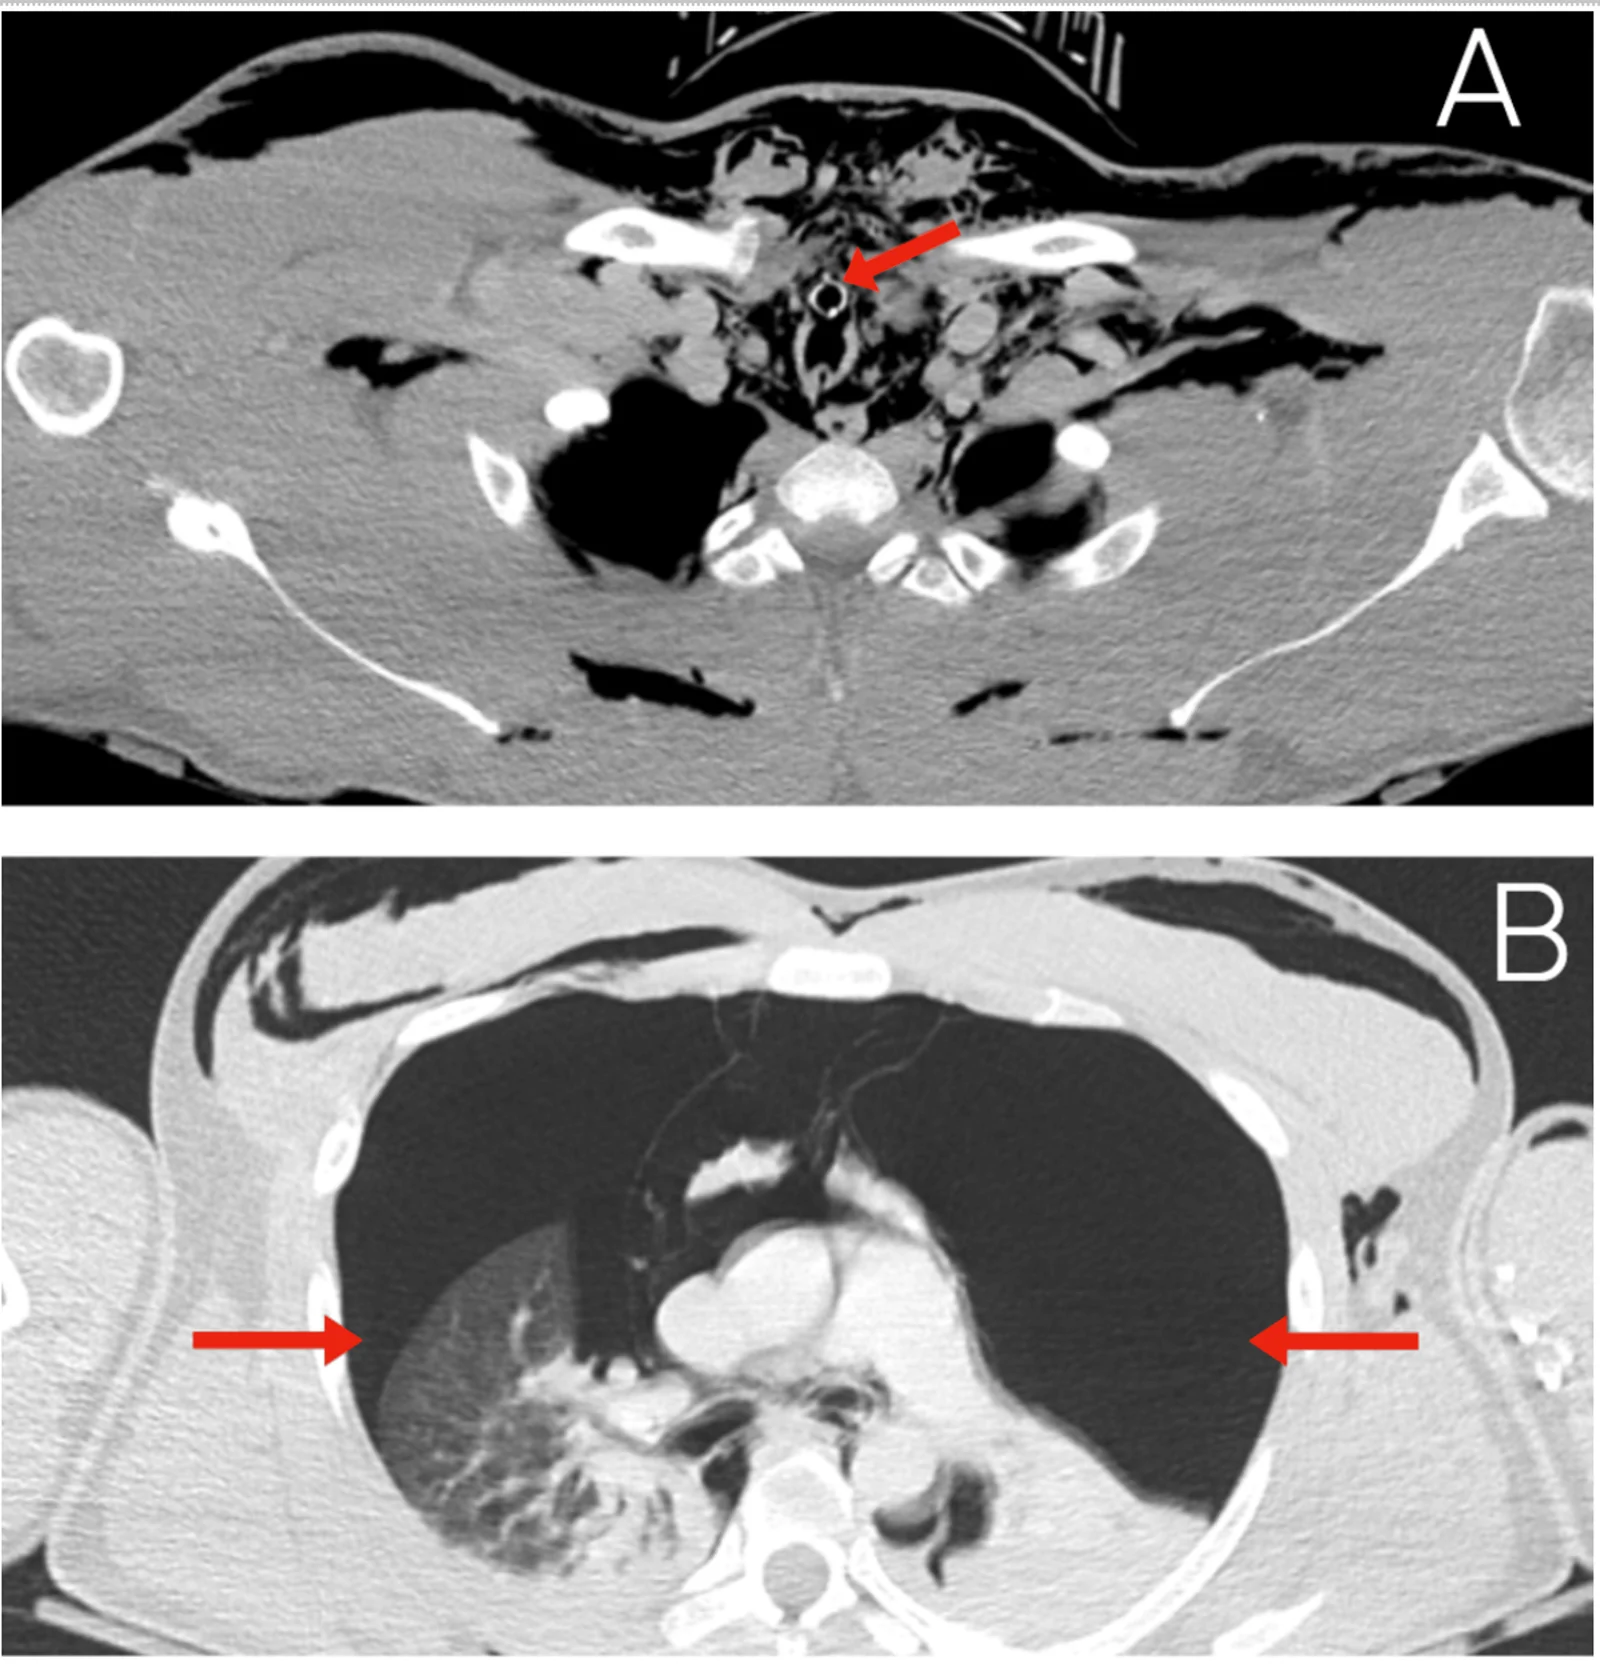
CT demonstrating traumatic airway injury and associated thoracic complications. A: CT of the neck showing rupture of the anterior tracheal wall with the endotracheal tube extending into the cervical soft tissues at the level of the superior border of the manubrium (red arrow); B: CT of the chest demonstrating large bilateral pneumothoraces with complete collapse of the left lung and partial collapse of the right lung (red arrows), along with extensive pneumomediastinum.
